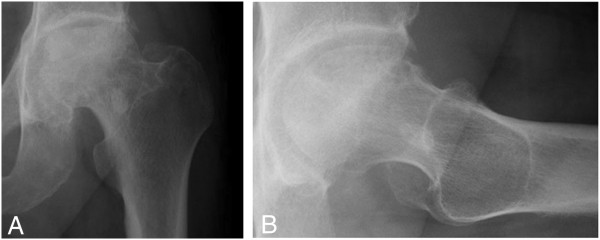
Plain radiographs show a fused acetabular fracture and collapsed left femoral head. (A) Anteroposterior and (B) lateral plain radiographs of the left hip obtained a year and a half after the left acetabular fracture show collapse in the anterosuperior portion of the left femoral head with joint space narrowing.
